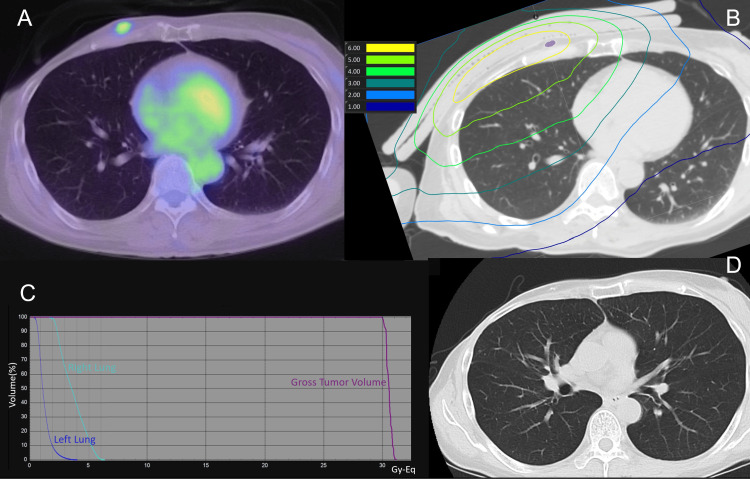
(A) Pre-treatment PET-CT. (B) Dose distribution map for BNCT (lung). Purple represents the GTV. (C) Dose-volume histogram. (D) CT scan 90 days post-treatment, showing a reduction in the GTV. BNCT: boron neutron capture therapy, GTV: gross tumor volume
